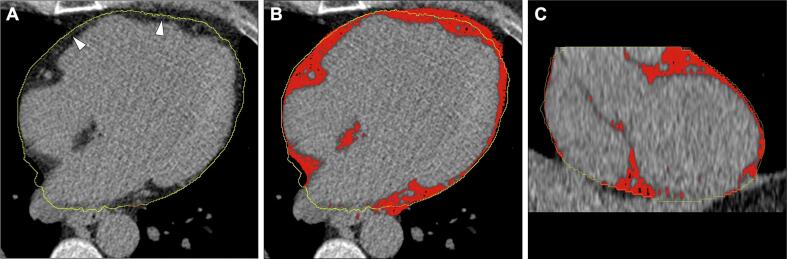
Cardiac CT scans in the axial plane without (A) and with red-colored overlays of fat (B), and in the coronal plane (C). The yellow tracings cover the pericardium (white arrowheads in A) and constitute the borders for EAT measurements.
